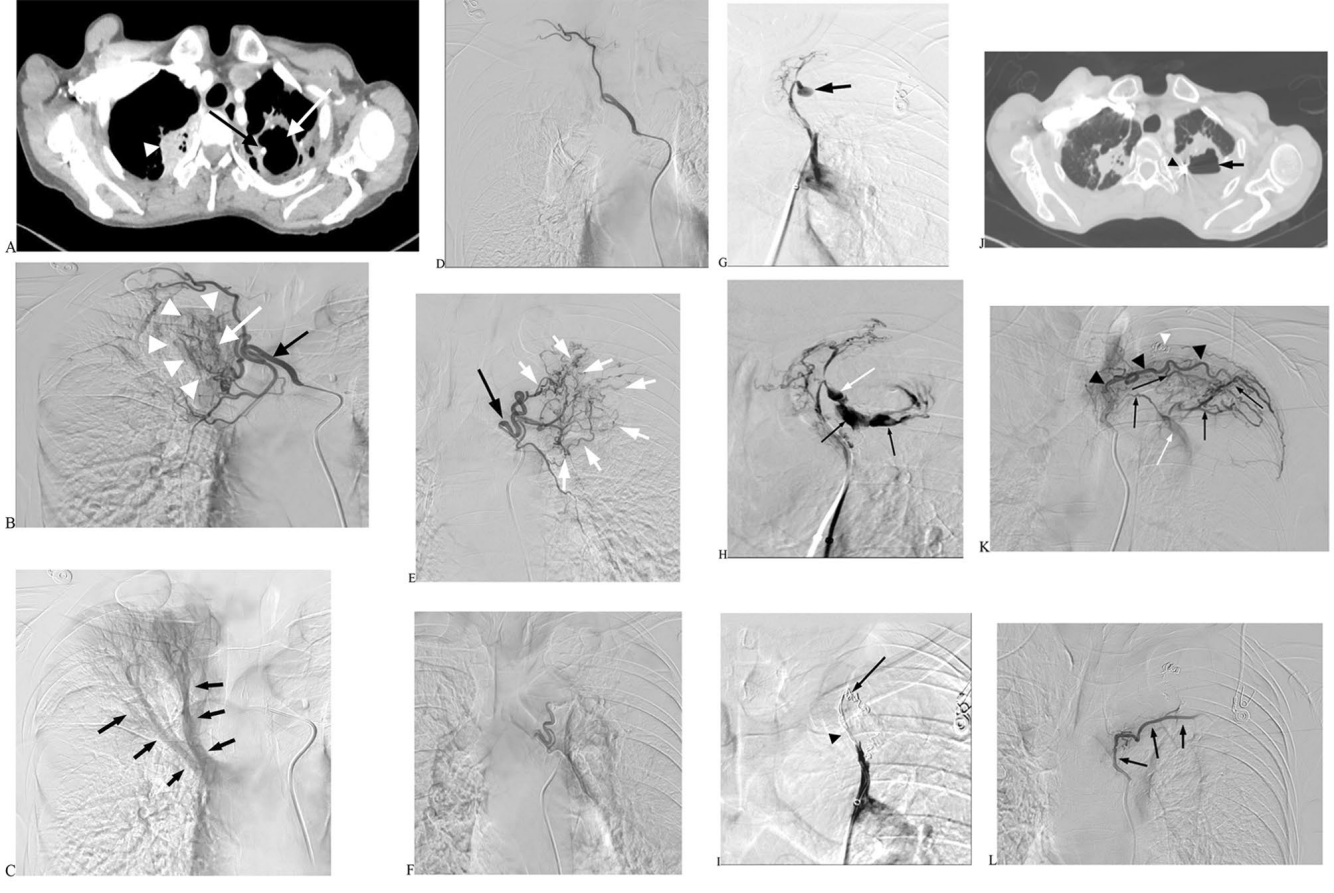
The patient with old pulmonary tuberculosis was hospitalized for 2 days with intermittent hemoptysis. The maximum hemoptysis volume in 24 h was approximately 700ml. A. Chest enhanced CT showing old lesions in the upper lobe of the right lung (white arrowhead). The left upper lobe cavity (white arrow) was formed, and PAP was observed in the cavity (black arrow). B. Right intercostal bronchial artery (black arrow) angiography shows obvious thickening, increased number, disorder, and structural abnormality of the bronchial artery (white arrowhead) and premature pulmonary artery branch (white arrow) in the upper lobe of the right lung. C. Right intercostal bronchial arteriography shows reverse flow in the late stage of the artery. The angiography also shows the pulmonary artery (black arrow) in the upper lobe of the right lung. D. After intercostal bronchial artery embolization with 350-560 μm PVA particles, the main intercostal artery remained intact. The bronchial artery and its branches disappeared completely, indicating complete embolization. E. Left bronchial artery (black arrow) angiography shows obvious thickening, increased number, disorder, and structural abnormality of the bronchial artery (white arrow). No obvious PA or PAP was observed. F. Left bronchial artery angiography again shows left BA branch vessels disappeared post BAE in 350-560 μm PVA particles. G. PAP showed on the left upper lobe of the lung by introducing a single curved catheter assisted by the long vascular sheath (black arrow) pulmonary angiography. H. PAP (white arrow) rupture, and contrast agent entering the cavity (black arrow) as observed in pulmonary angiography. I. Angiography after PAPE + PAE with micro coils, dense embolization with micro coils in parent artery (black arrowhead), and displacement of the micro coils at PAP rupture (black arrow). J. The patient was discharged from the hospital and admitted again with hemoptysis 13 months later. The amount of hemoptysis was about 100ml within 24 h. Enhanced chest CT examination showed that the cavity in the upper lobe of the left lung (black arrow) was smaller than before, and the shadow of the micro coils was visible within it (black arrowhead). K. Left intercostal artery (black arrowhead) angiography shows a large number of collateral vessels (black arrows) deviating into the lung, forming a large number of tortuous, thickened, malformed vessels, pulmonary artery fistula (white arrow), and micro coils shadow (white arrowhead). L. The presence of the main intercostal artery (black arrow) and the disappearance of collateral malformed vessels and pulmonary artery fistula were angiographed with 350-560 μm PVA embolization
